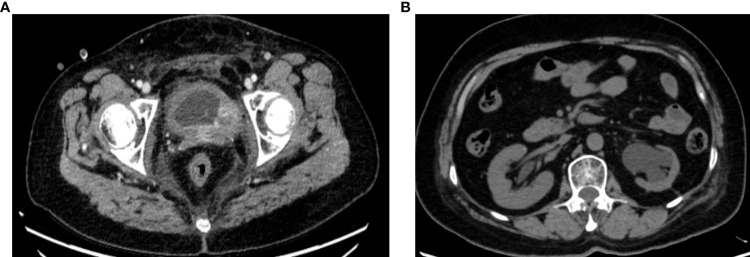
(A, B) Urinary tract computed tomography urography (October 24, 2021).
